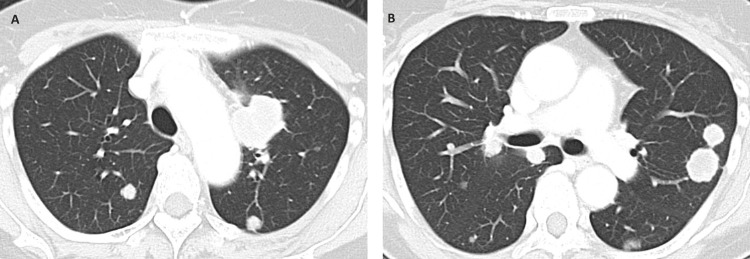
CT scan images of the chest one year after radiotherapy There were multiple nodules in both (A and B) lungs, and the diagnosis was multiple pulmonary metastases.
